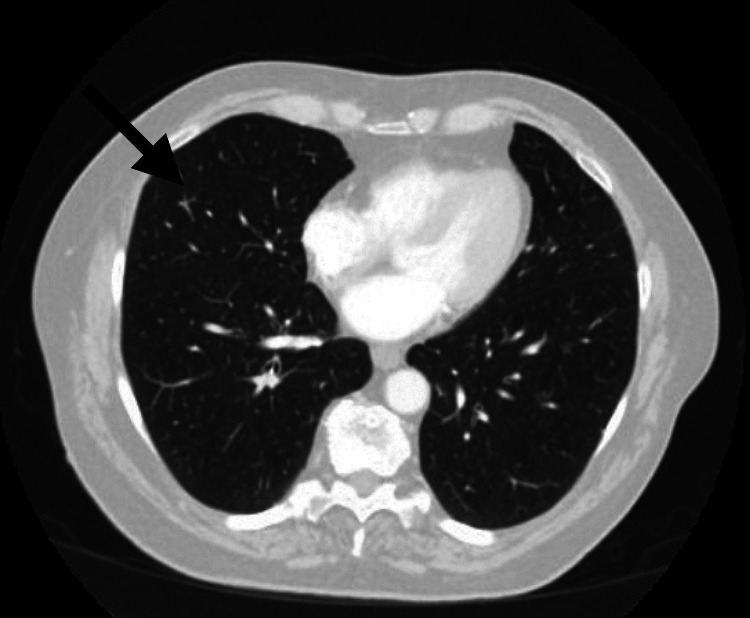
CT of the chest showing evidence of resolving right middle lobe nodules (previously seen in the location indicated with an arrow) after radiation to the chest wall and before pembrolizumab therapy
